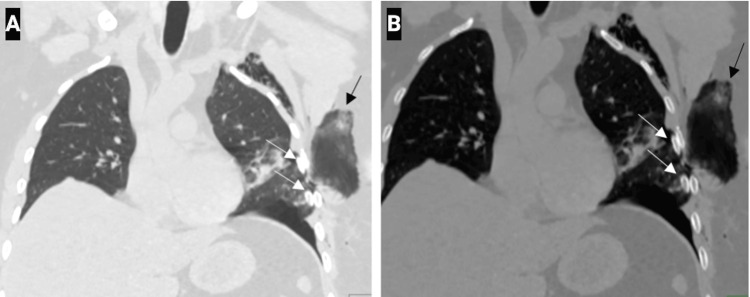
Coronal chest CT image with lung window (A) and bone window (B) setting shows lung herniation through a defect (black arrow) between the linear displaced fractures of the left fourth and fifth ribs (white arrows). Subcutaneous emphysema is also seen.
